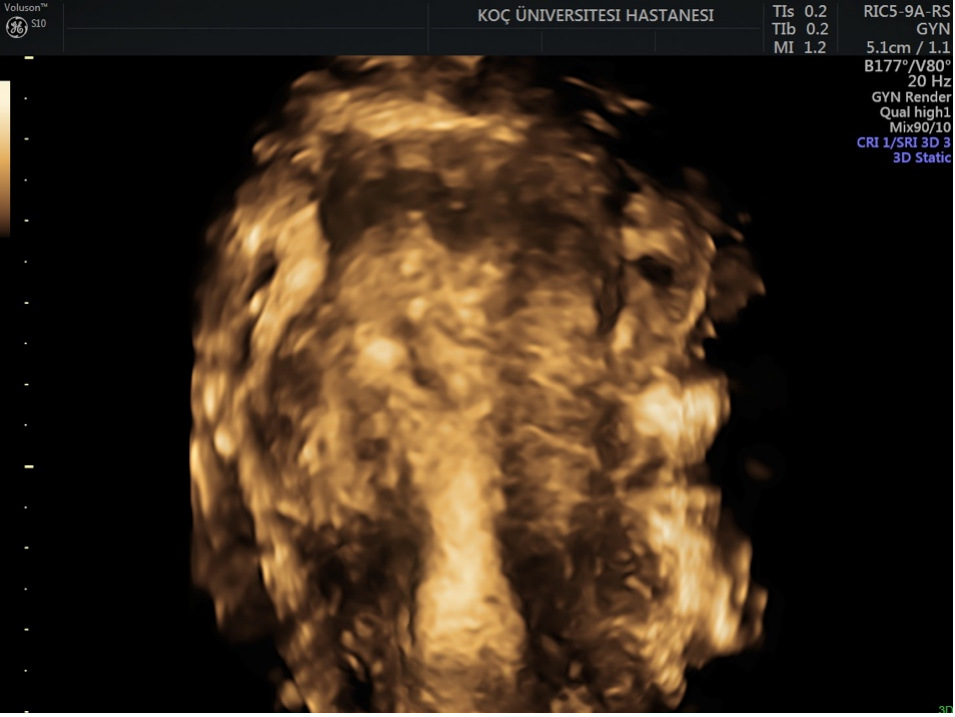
Postoperative 3D ultrasound image of a patient who underwent laparoscopic adenomyomectomy erroneously diagnosed as fibroid before surgery. Note the large myometrial defect on the right cornual portion and irregular endometrial cavity with multiple adhesions. Also, note the enlarged and irregular junctional zone on the fundal region suggesting presence of adenomyosis.
